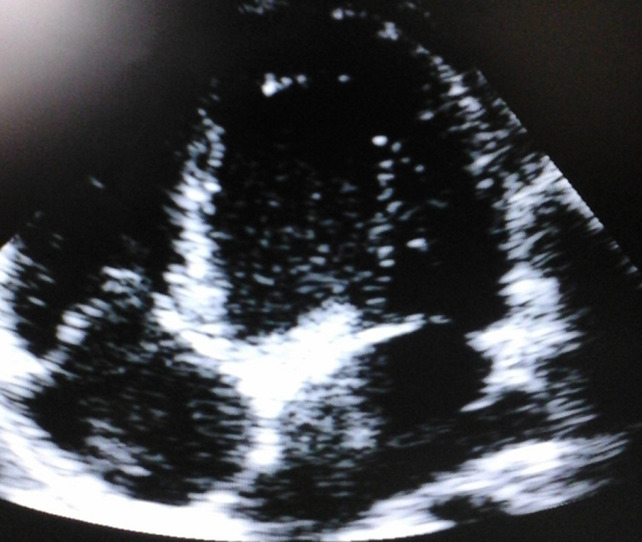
apical four chambers view showing the absence of re-accumulation of pericardial effusion
